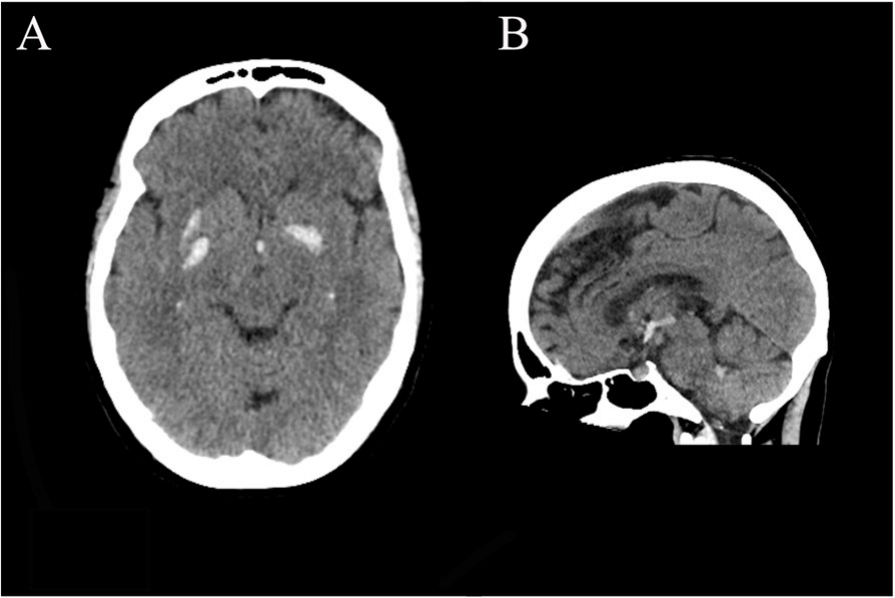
A Axial and (B) sagittal non-contrast computed tomography images show irregular hyperdensities along the right basal ganglia, contiguous along the anterior commissure (AC) and extending into the contralateral globus pallidus. Additional blood products are noted predominantly in the third ventricle and to a lesser extent in the fourth ventricle. Images acquired with a Philips Brilliance ICT 256 slice CT scanner
